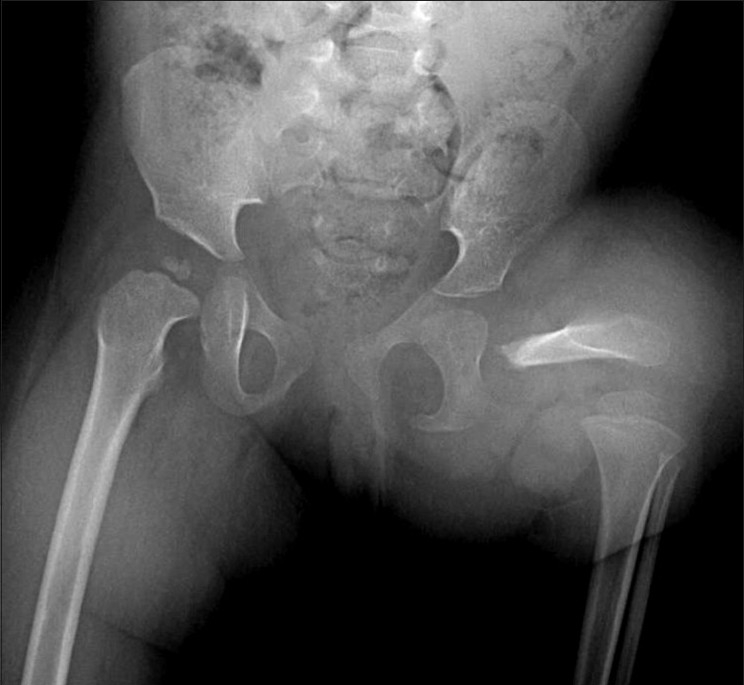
Proximal focal femoral deficiency: Frontal radiograph of the pelvis and both hips shows that the proximal femur is absent and the acetabulum is malformed on the left side. Only a hypoplastic portion of the distal left femur is apparent
